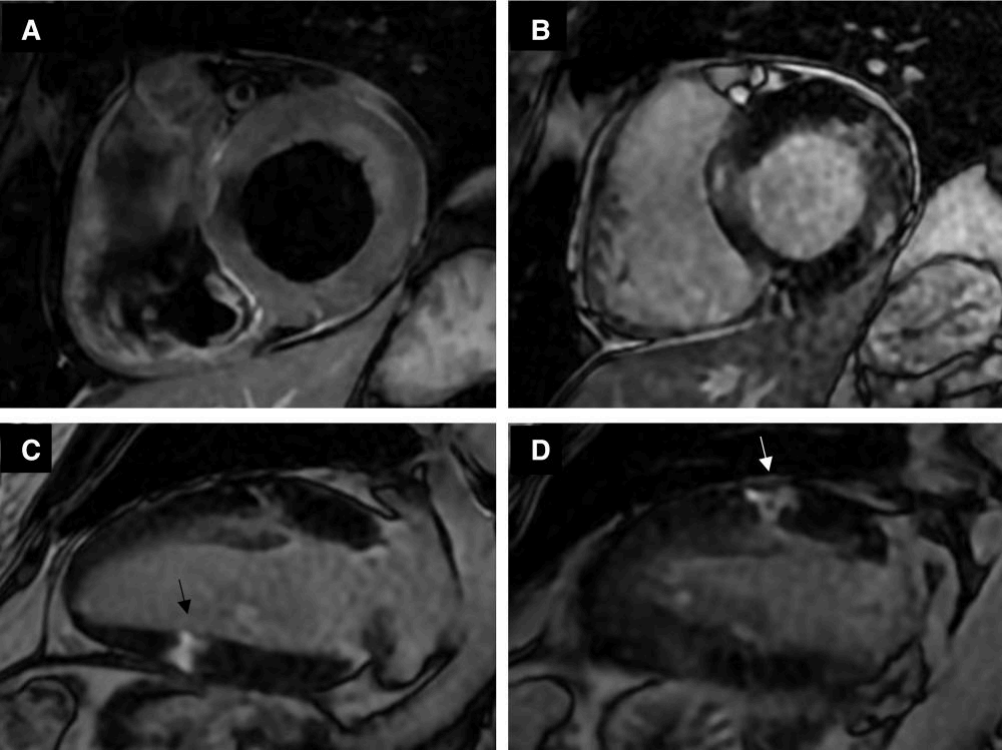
CMR, multifocal ischaemic changes. CMR T2-weighted image (*A*) and myocardial delayed enhancement images (*B–D*) showing ischaemic changes in the left anterior descending and circumflex artery territories.
